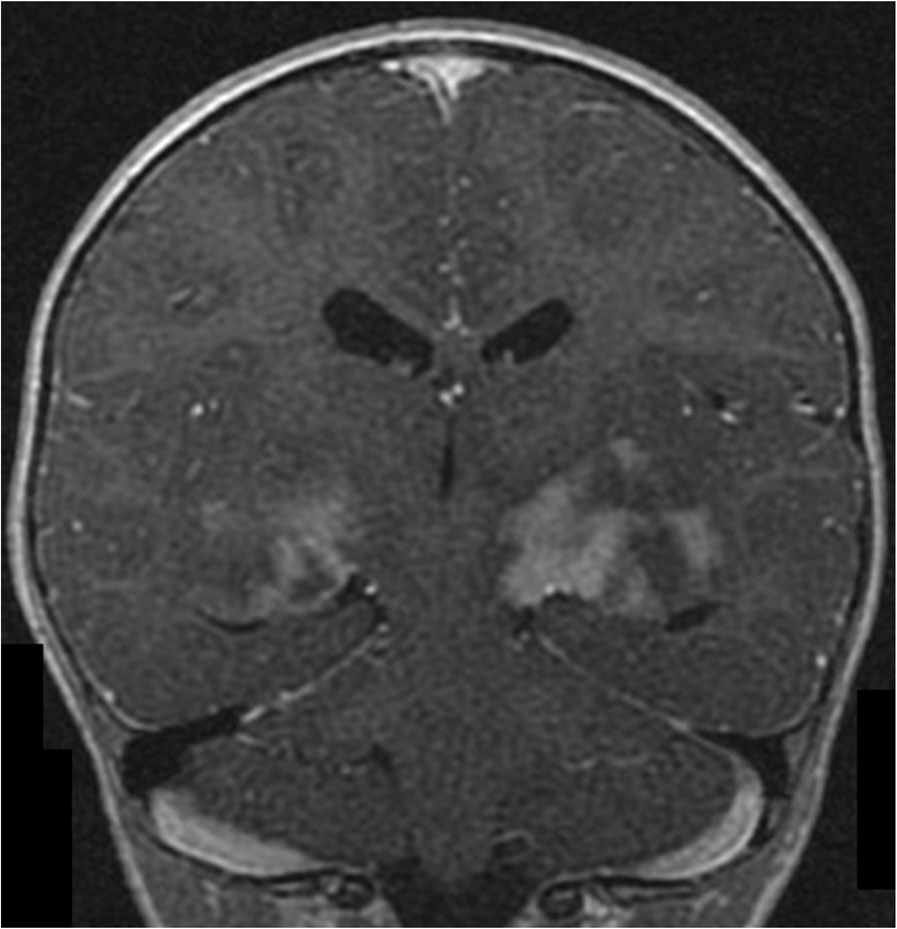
A coronal T1-weighted brain MRI of a 22 months old female with NF1 is shown. There is heterogeneous contrast enhancement involving the basal ganglia and internal capsule bilaterally
